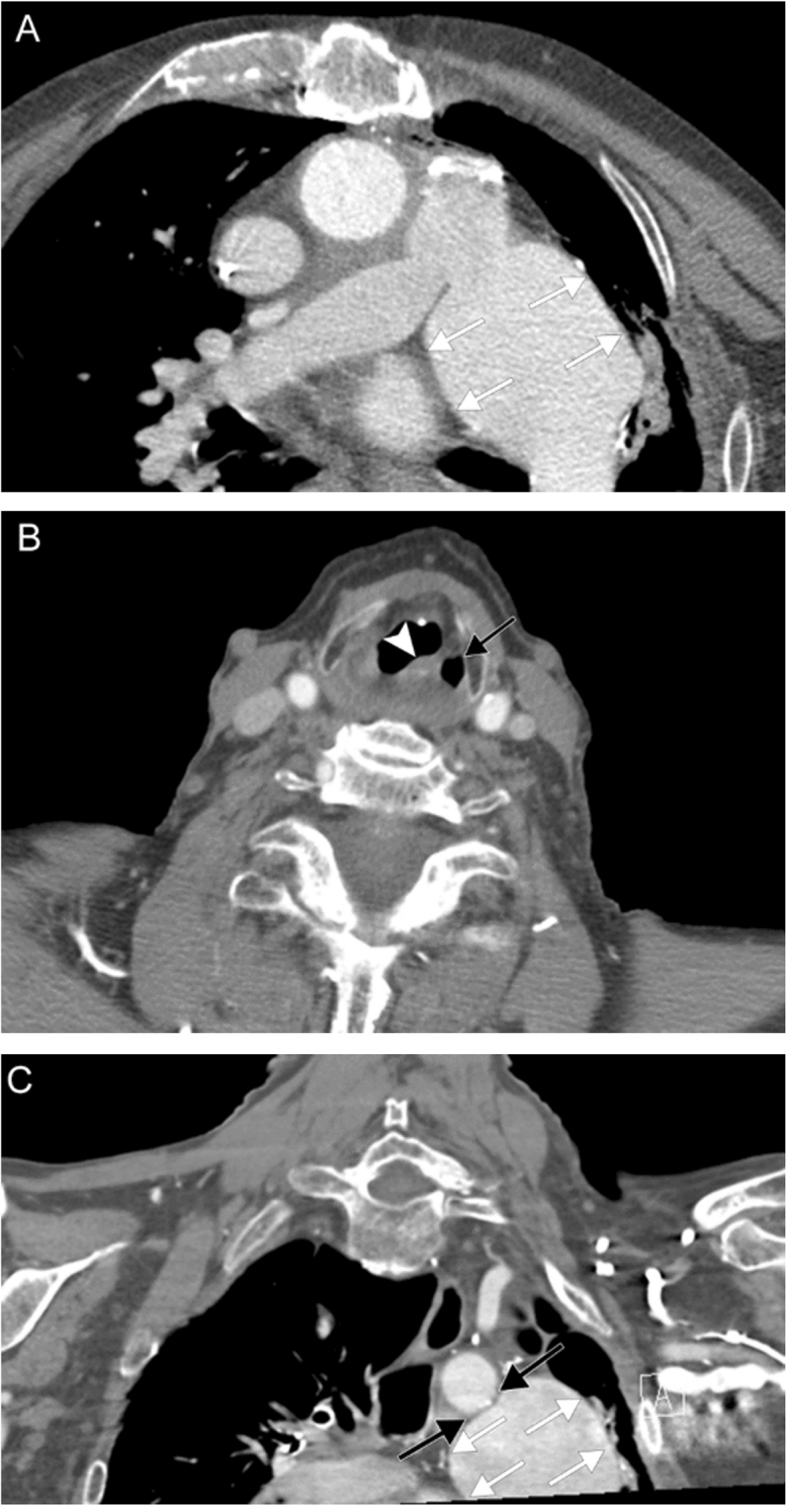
Pulmonary artery aneurysm. A 78-year-old man with advanced congestive heart failure secondary to congenital pulmonary artery stenosis status post multiple corrective surgeries, now presenting with progressive shortness of breath and speaking difficulty despite adequate management of heart failure. Axial CTA image (a) through the mediastinum reveals aneurysmal dilatation of the left pulmonary artery (white arrows). An axial CTA image through the larynx (b) reveals thickening of the left aryepiglottic fold (white arrowhead) and dilatation of the left piriform sinus (black arrow). A coronal CTA image (c) demonstrates effacement of the aortopulmonary window (black arrows) by the pulmonary artery aneurysm (white arrows) with presumed compression of the left recurrent laryngeal nerve
